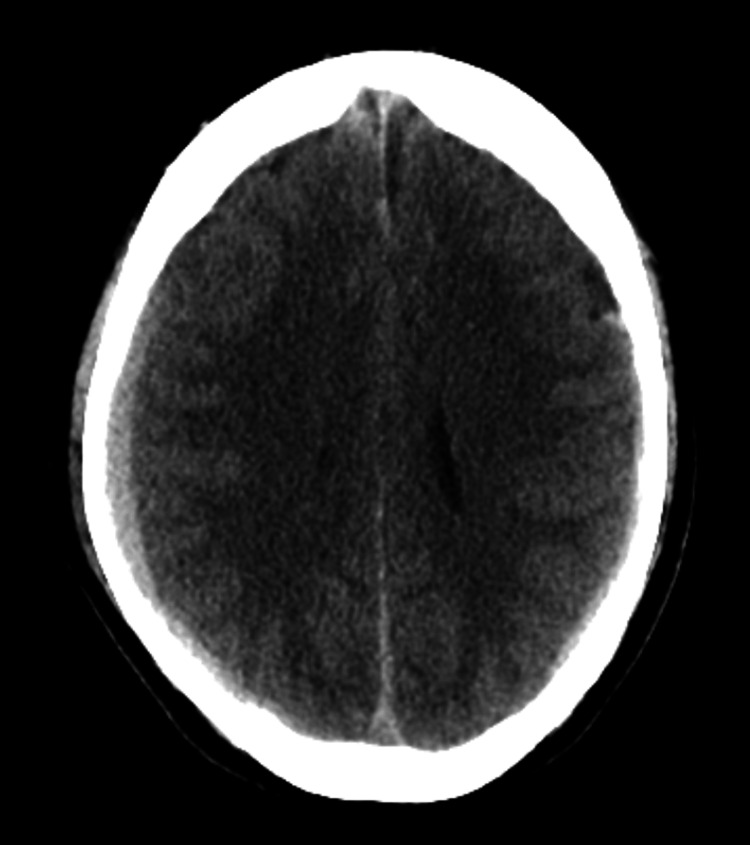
CT head at initial presentation displaying 9 mm right and 3 mm left acute-on-chronic subdural hematomas, with a 1 mm leftward midline shift
